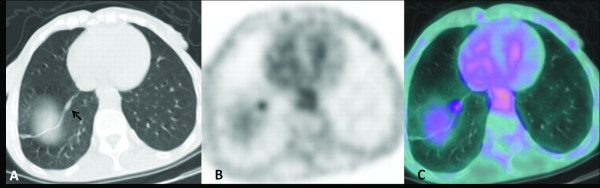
The undetermined nodule. ^18^ F-FDG PET-CT: CT (a), PET (b), and fused (c) axial images. A focus of intense ^18^ F-FDG uptake was evident in the lower right lobe corresponding to the nodule located in the context of an atelectatic band (black arrow).
